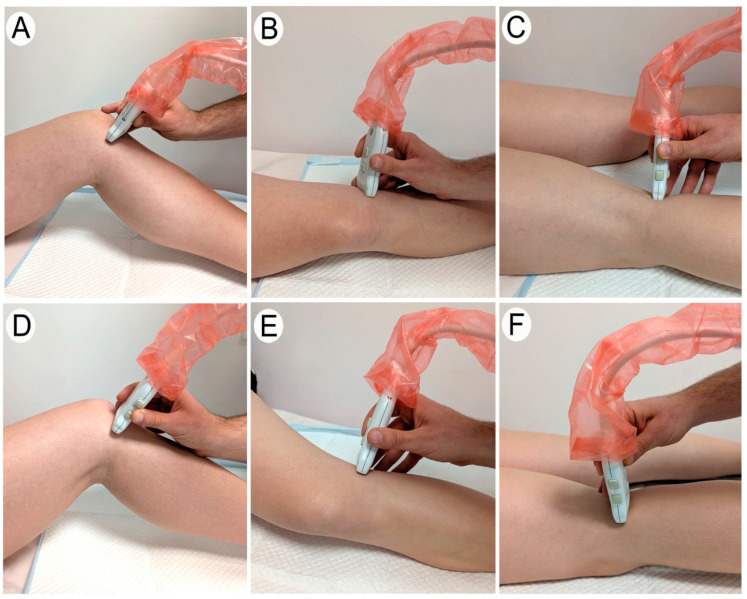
A longitudinal ultrasound scan of the medial and lateral meniscus. The images show the appropriate transducer placement to obtain a longitudinal view of each meniscal region. (A–C) Longitudinal ultrasound imaging of the medial meniscus: the anterior (A), middle (B), and posterior (C) regions. (D–F) Longitudinal ultrasound imaging of the lateral meniscus: the anterior (D), middle (E), and posterior (F) regions.
